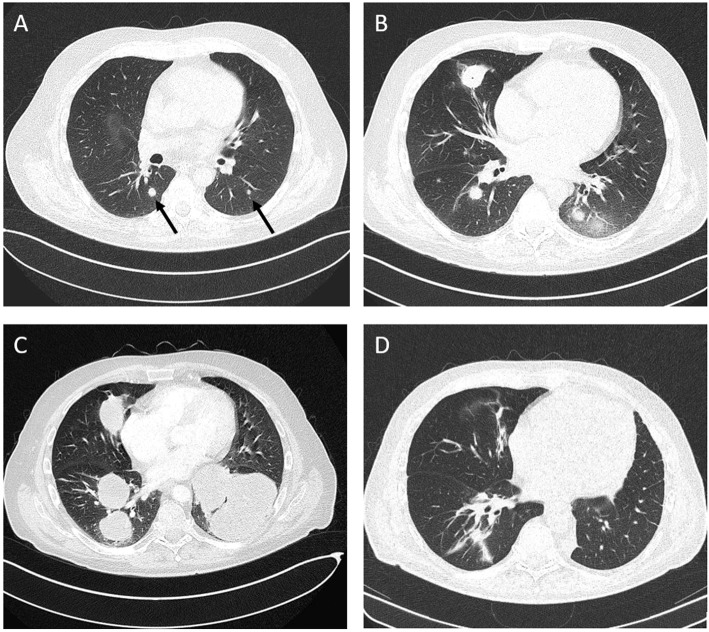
Computed tomography (CT), chest. (A) Disseminated atypical pulmonary infiltrates (day +145 after alloHSCT), (B) Chest CT following antimycotic therapy (day +170), (C) Disease progression after three courses of rituximab and two courses R-CHOP (day +228), (D) Complete remission (CR) after five courses of brentuximab vedotin and three courses of third-party EBV-specific T-cells.
